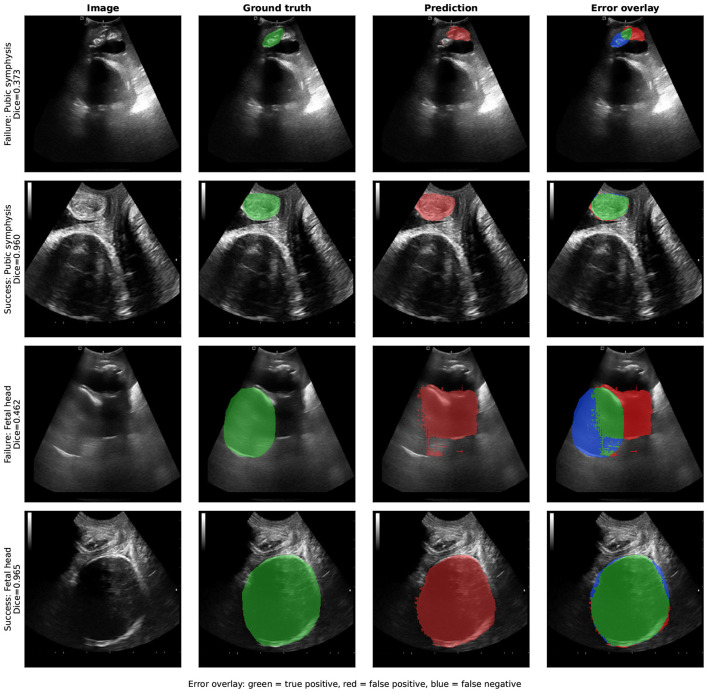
Fixed-rule PSFHS qualitative analysis. For each foreground class, the lowest- and highest-Dice test records from F-TPA-SAM2 (*N* = 400, seed 1) are shown. Error overlay: green = true positive, red = false positive, blue = false negative. The selection rule was fixed before visual inspection and is separate from the wrong-class prompt analysis in Figure 8. The fetal ultrasound images in Figure were adapted from the PSFHS dataset [Lu et al. (23); Zenodo record 10969427; CC BY 4.0].
